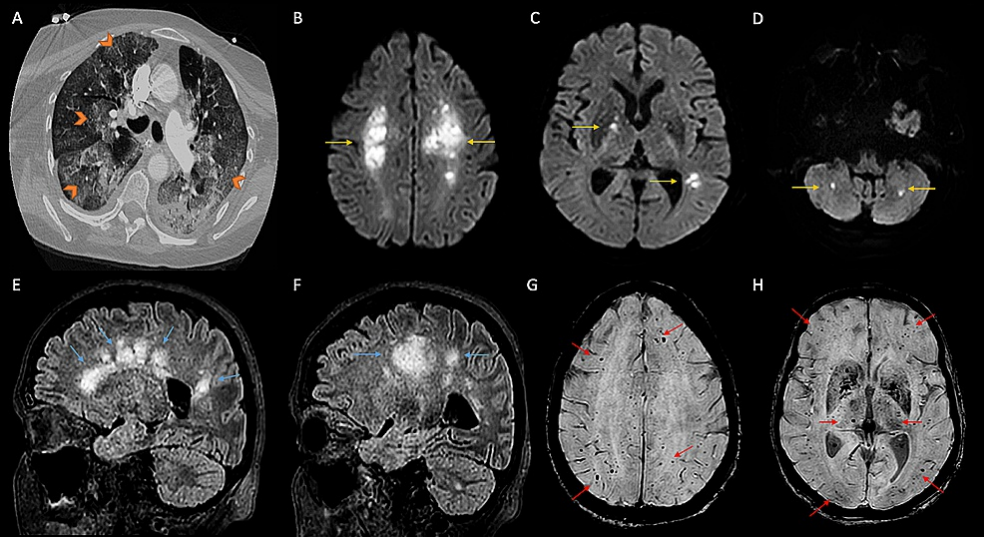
Imaging findings of patient 2 (A) CT (axial section) of the chest with contrast showing diffuse bilateral subpleural and perihilar ground-glass opacities (orange arrowheads). (B-D) MRI of the brain (diffusion-weighted imaging, axial sections) showing hyperintensities in bilateral centrum semiovale, basal ganglia, and bilateral cerebellar hemispheres (yellow arrows). (E, F) T2/FLAIR sagittal images showing confluent hyperintensities in periventricular regions, centrum semiovale in frontoparietal areas, and cerebellum (blue arrows). (G, H) Susceptibility-weighted imaging showing multiple foci of microhemorrhages in cortical and subcortical regions, and bilateral basal ganglia (red arrows). CT, computed tomography; MRI, magnetic resonance imaging; T2/FLAIR, T2-weighted/fluid-attenuated inversion recovery
